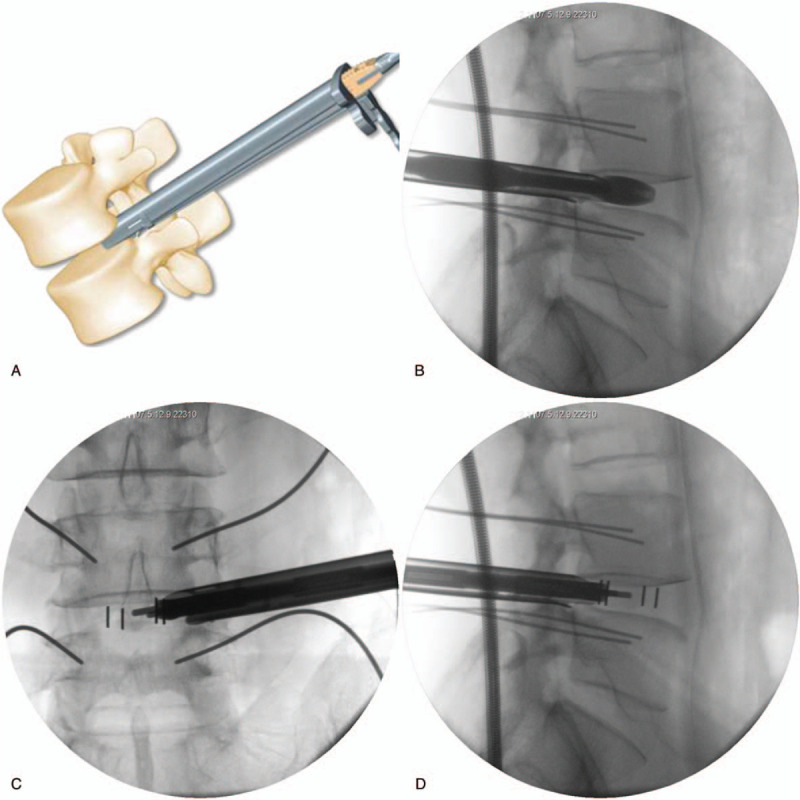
According to the test model (A and B), the appropriate size of the cage was selected, and the position of the cage was examined by anteroposterior (C) and lateral (D) radiographs.
